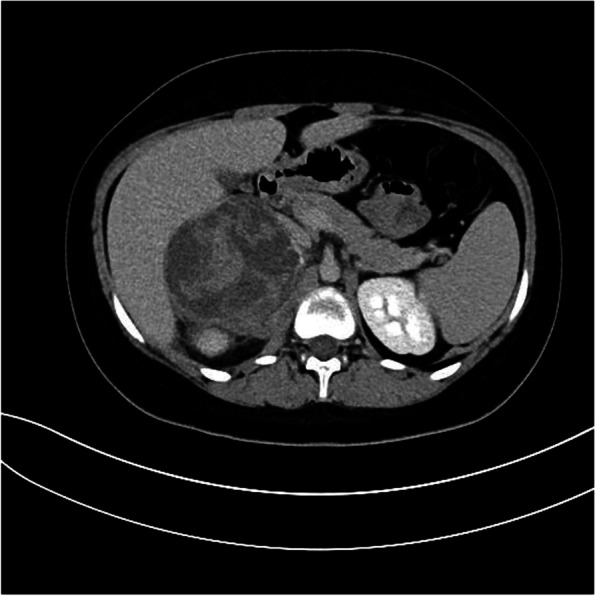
Contrast-enhanced computed tomography (CT), venous phase, axial view
